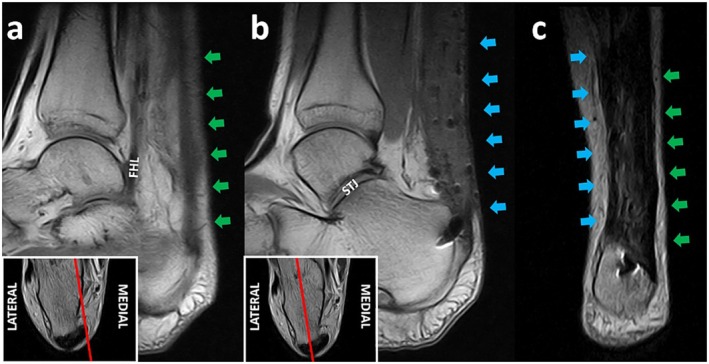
The 5 months MRI shows on sagittal plane the continuity of the most medial and superficial fibers (green arrows) with their intact native insertion (a) and the enlarged lateral and deep fibers (blue arrows) surgically reinserted to calcaneal tuberosity (b). Similar aspect is seen on the coronal plane (c).
